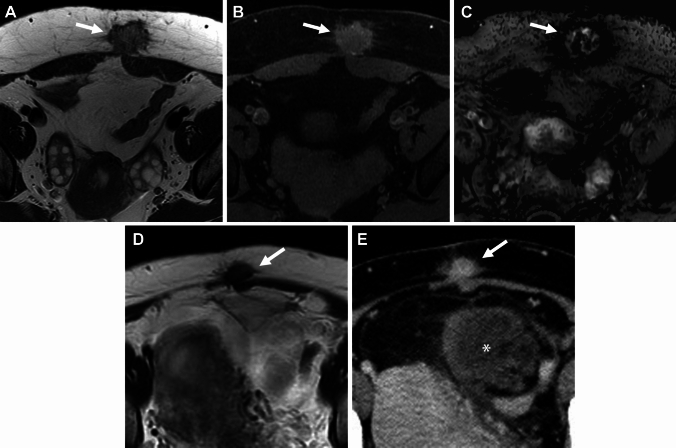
Abdominal wall endometriosis. A 34-year-old with lower abdominal discomfort during menstruation. A On T2-weighted image, a subcutaneous mass with irregular margins (arrow) exhibits heterogeneous signal intensity at the previous cesarean surgical scar. Small high signal intensity hemorrhagic foci are revealed on B fat-saturated T1-weighted image. Prominent signal voids are observed in and around the mass (arrow) on C SWI. A 37-year-old with lower abdominal discomfort during menstruation. D On T2-weighted image a subcutaneous low signal intensity mass (arrow) at the previous cesarean surgical scar is revealed. E On CT, linear infiltration irradiating peripherally from a central soft tissue mass (arrow) as the gorgon sign is observed. *: Concomitant mesenteric liposarcoma
